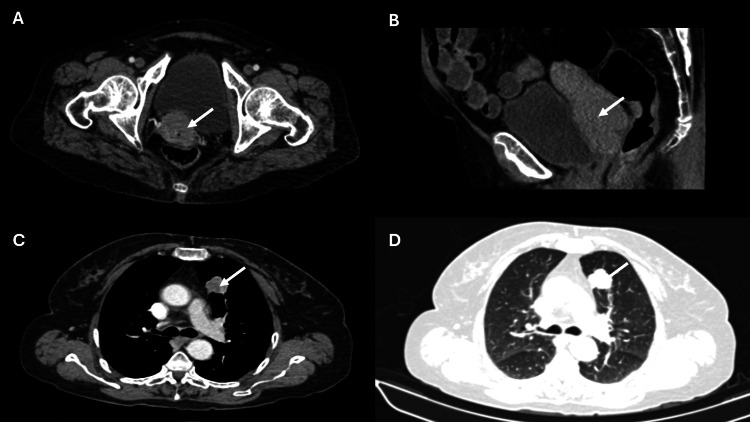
Cross-sectional (A) and sagittal (B) CT images reveal enhancing soft tissue lesions involving the cervix (white arrows). Axial images (C & D) reveal enhancing nodular soft tissue lesions in the anterior segment of the left lung upper lobe (white arrows).
